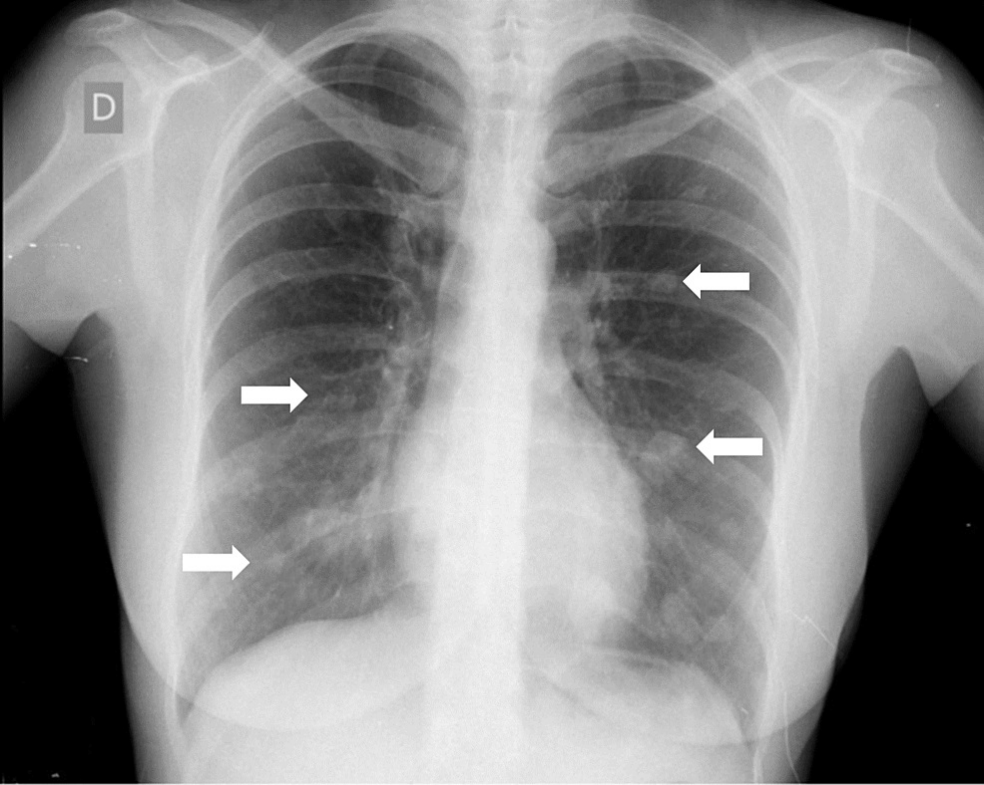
Chest radiograph showing bilateral pulmonary nodules (arrows)
